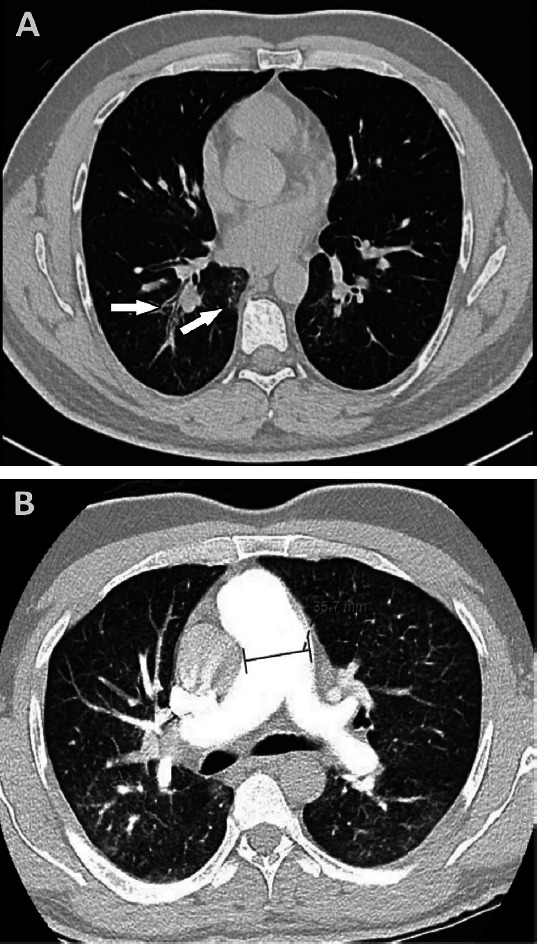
High resolution computed tomography scan of the chest. (A) Bilateral faint ground glass opacities with tree-in-bud appearance and mild bronchial wall thickening, more pronounced at the right lower lobe; (B) The main pulmonary trunk is enlarged by 3.3 cm.
